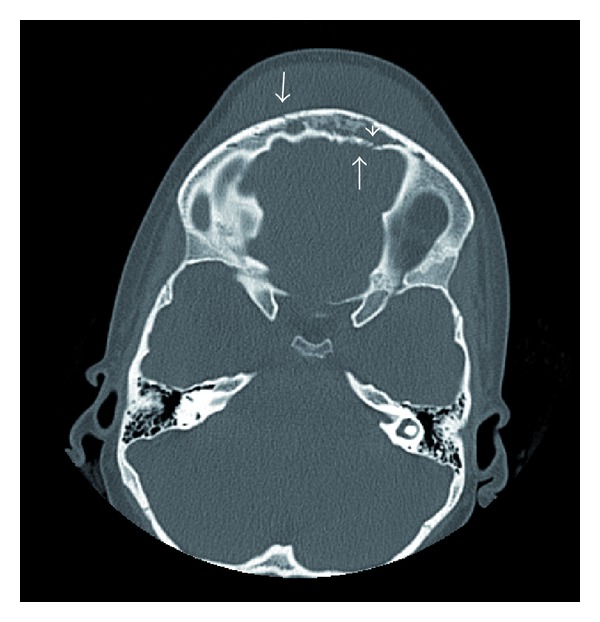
Axial CT without contrast on presentation demonstrating erosion of the anterior and posterior tables of the frontal sinus (arrows), frontal sinus opacification (arrowhead), and soft tissue swelling of the forehead.
